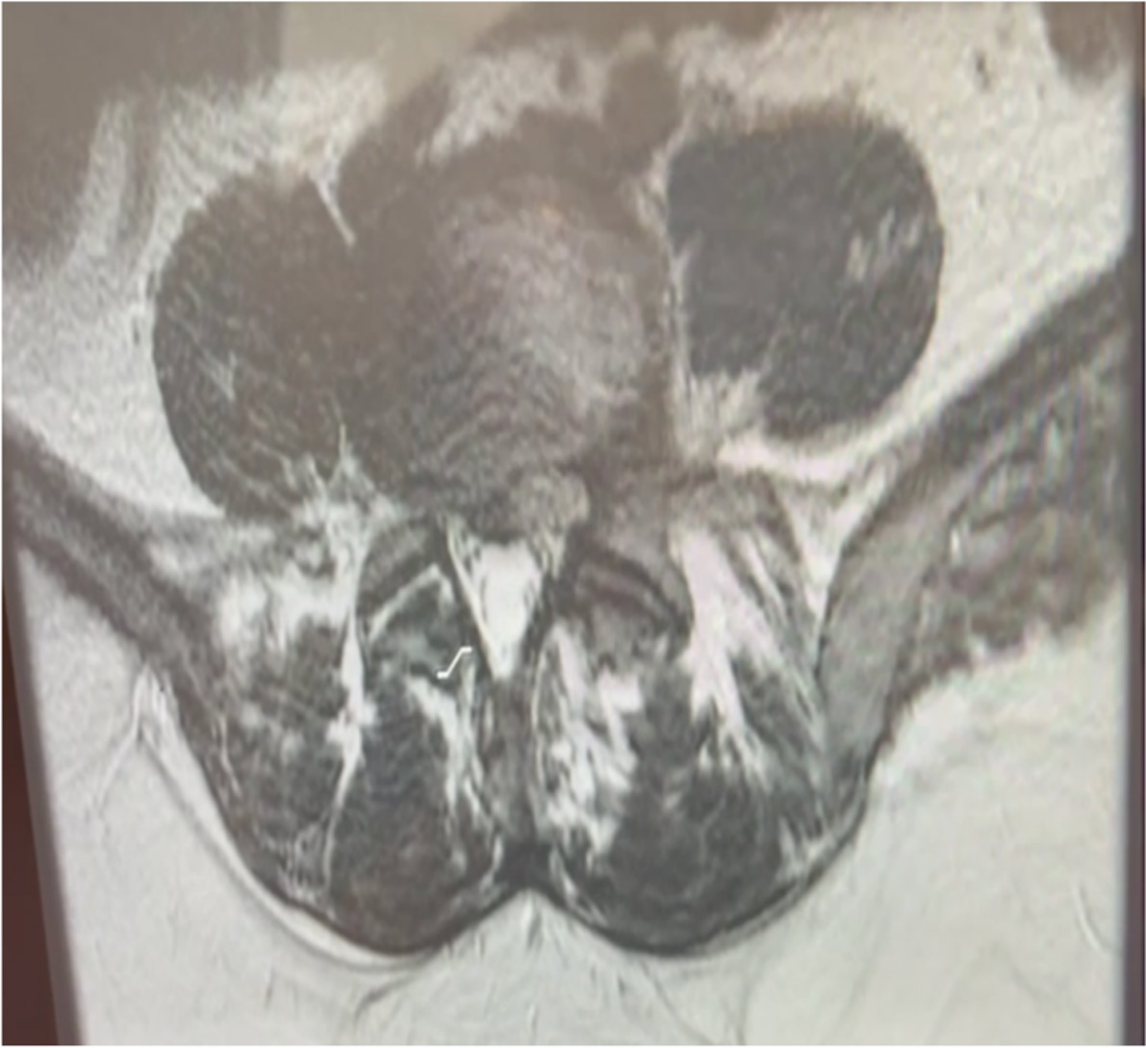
Axial MRI view of L5‐L4 before treatment
